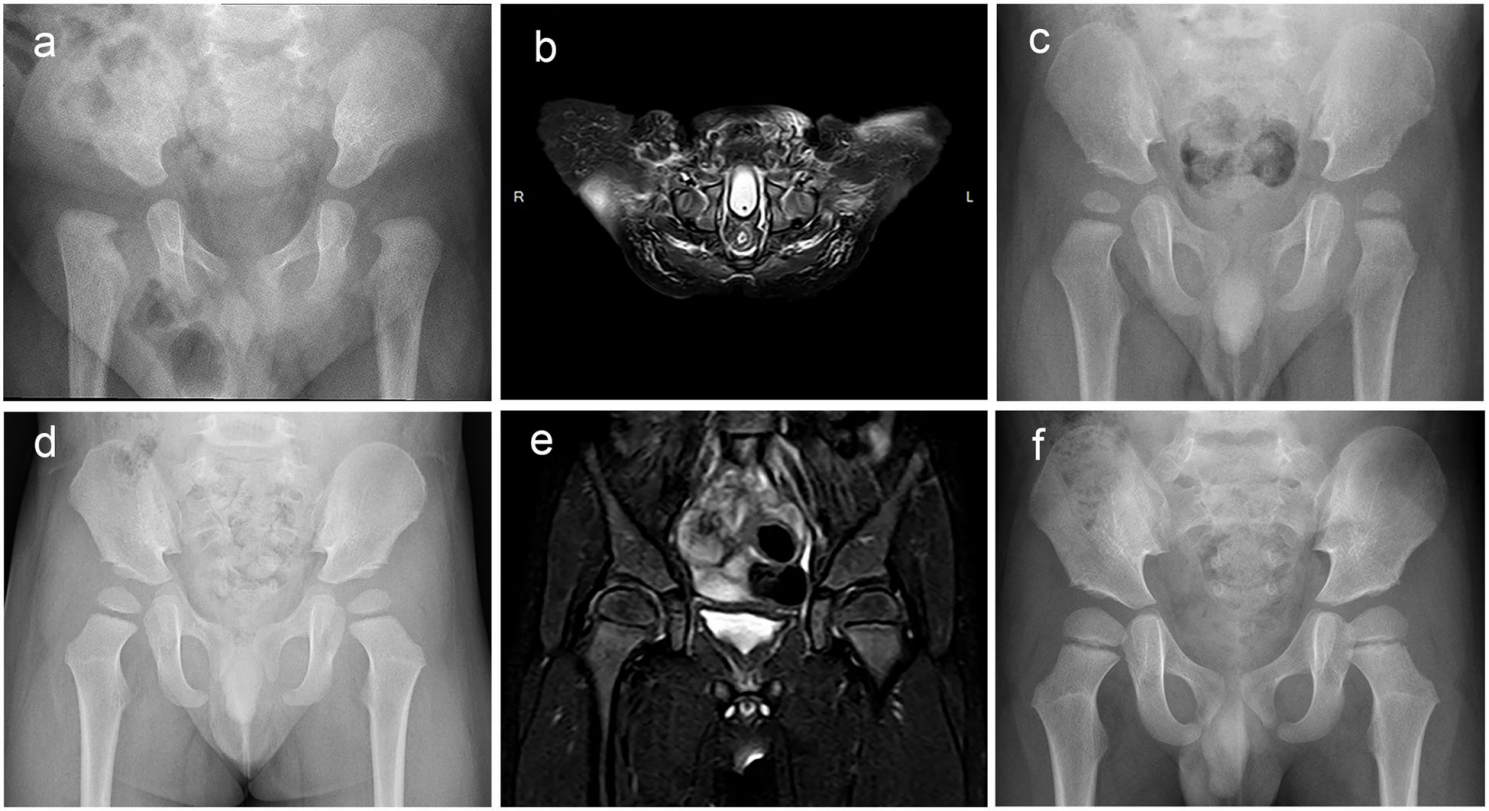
a Preoperative pelvic anteroposterior view in a five months old patient. b Immediate MRI after surgery confirmed satisfactory hip reduction. c and d Radiograph at 16 months and 26 months after open reduction, respectively. e MRI assessment at 55 months after open reduction. f Radiograph at 55 months after open reduction
